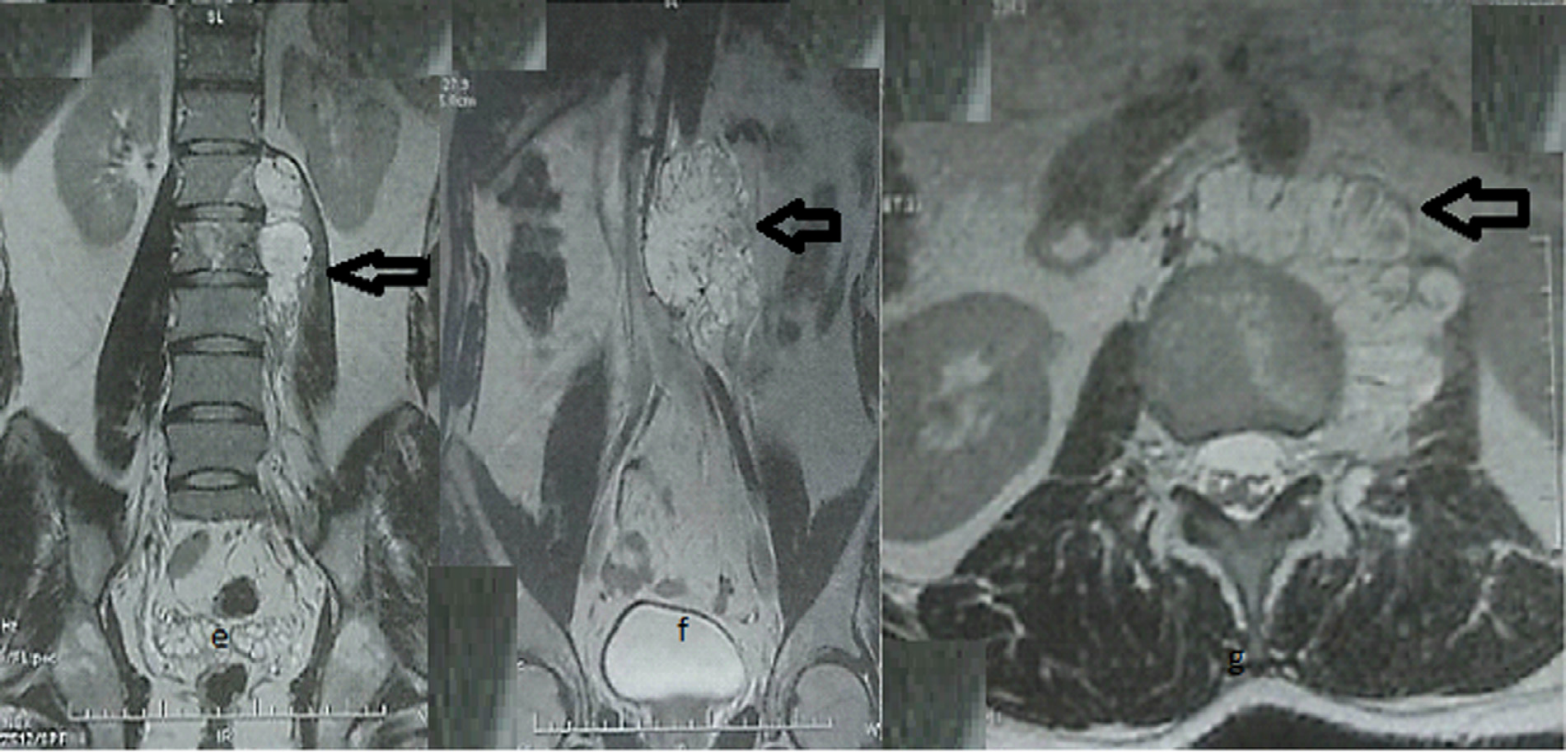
(E, F) T2-weighted coronal MRI of abdomen shows malignancy expanding into the abdomen and psoas muscle. (G) T2-weighted axial MRI of lumbar spine highlighting borders of tumor infiltrating posteriorly into the paraspinal musculature.
